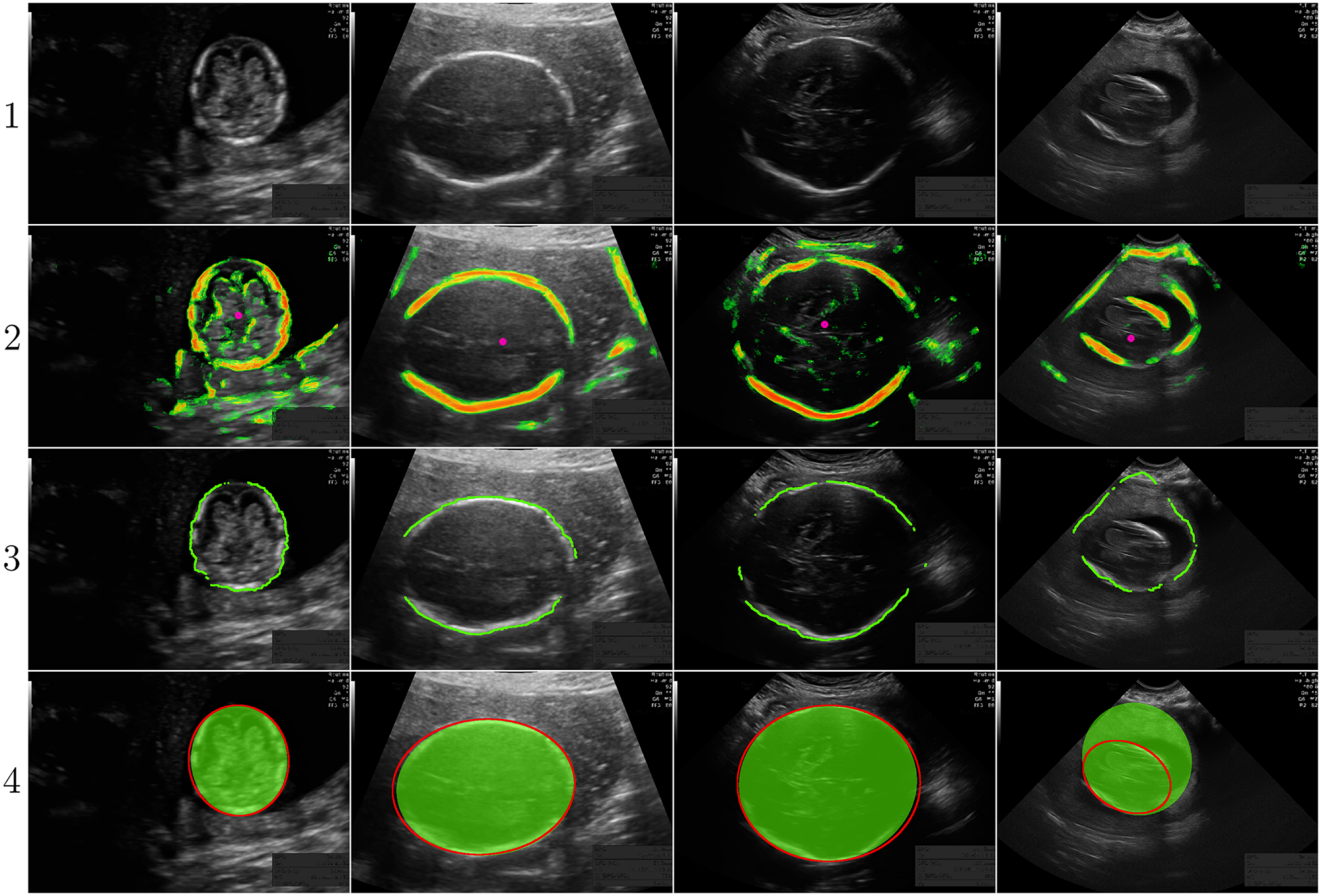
Results of quantification system *C* closest to the median ADF of system *C*. From left to right: first trimester with a ADF of 1.8 mm, result second trimester with a ADF of 1.6 mm, result third trimester with an ADF of 4.2 mm and worst result first trimester with an ADF of 36.8 mm. From top to bottom: (1) The ultrasound image. (2) The ultrasound image with overlay of the pixel classifier likelihood ranging from green to red and the Hough transform result in pink. (3) The ultrasound image with an overlay of the highest fifth percentile repositioned dynamic programming pixels. (4) The ultrasound image with the fitted ellipse in green and the annotation of observer 1 in red.
